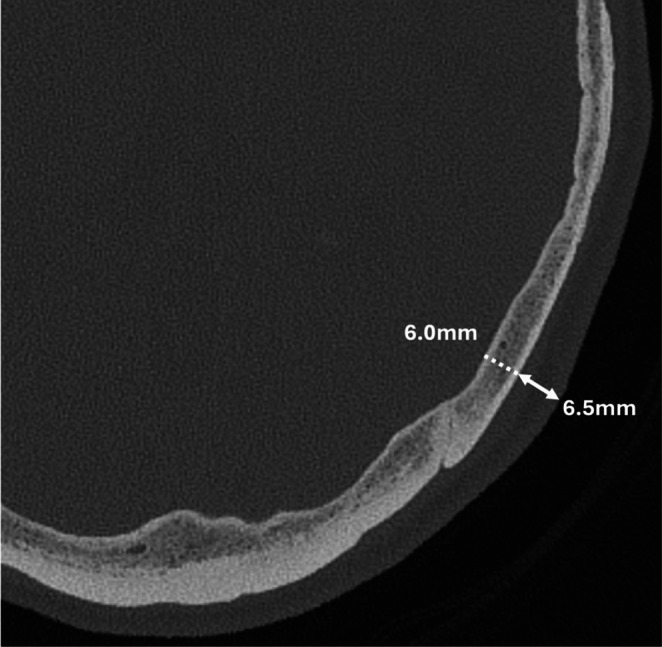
Scalp and calvarial thickness. Axial CT demonstrating measurement of scalp thickness (double arrow) and calvarial thickness (dashed line). These parameters are important for incision planning, secure fixation of the receiver well, and avoid dural injury
